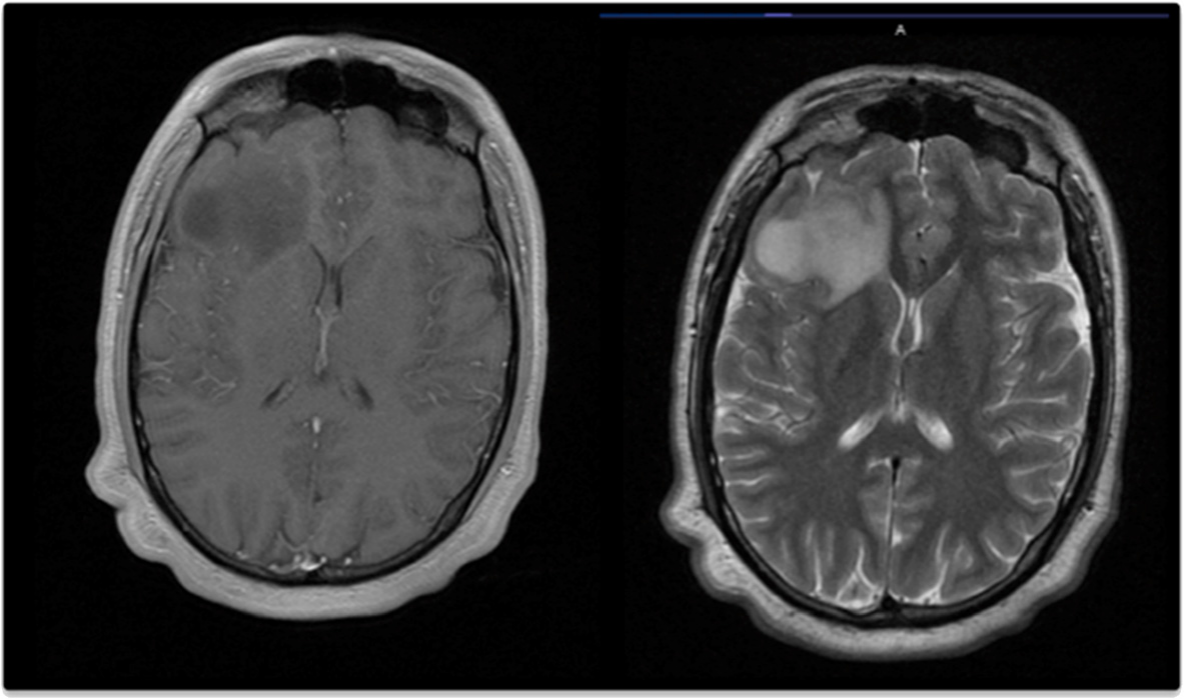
Brain magnetic resonance imaging (MRI): T1 gadolinium sequence (left) and T2 sequence (right).
